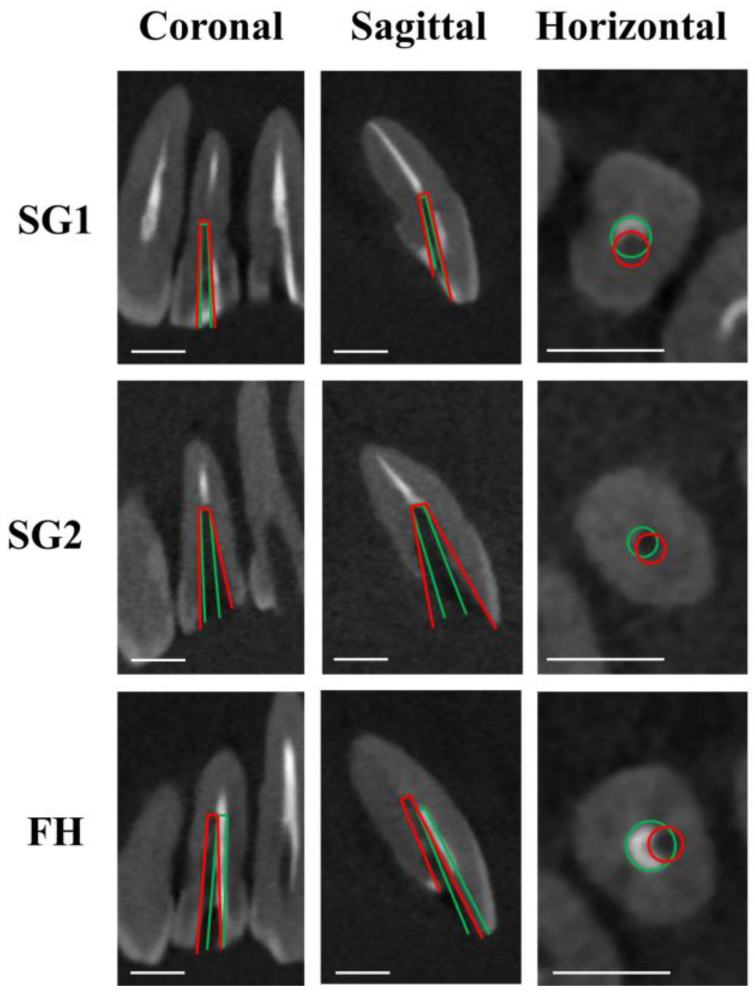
Representative CBCT images after fiber post removal on lateral incisors. Green lines show planned pathways, and red lines show actual drilling pathways. Scale bar = 5 mm.
